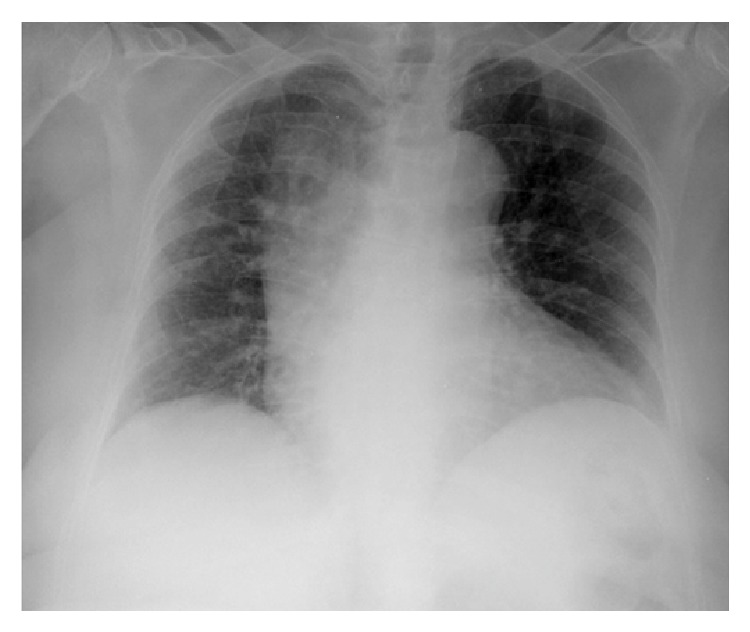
Chest X-ray on admission: right upper lobe alveolar infiltrate.
